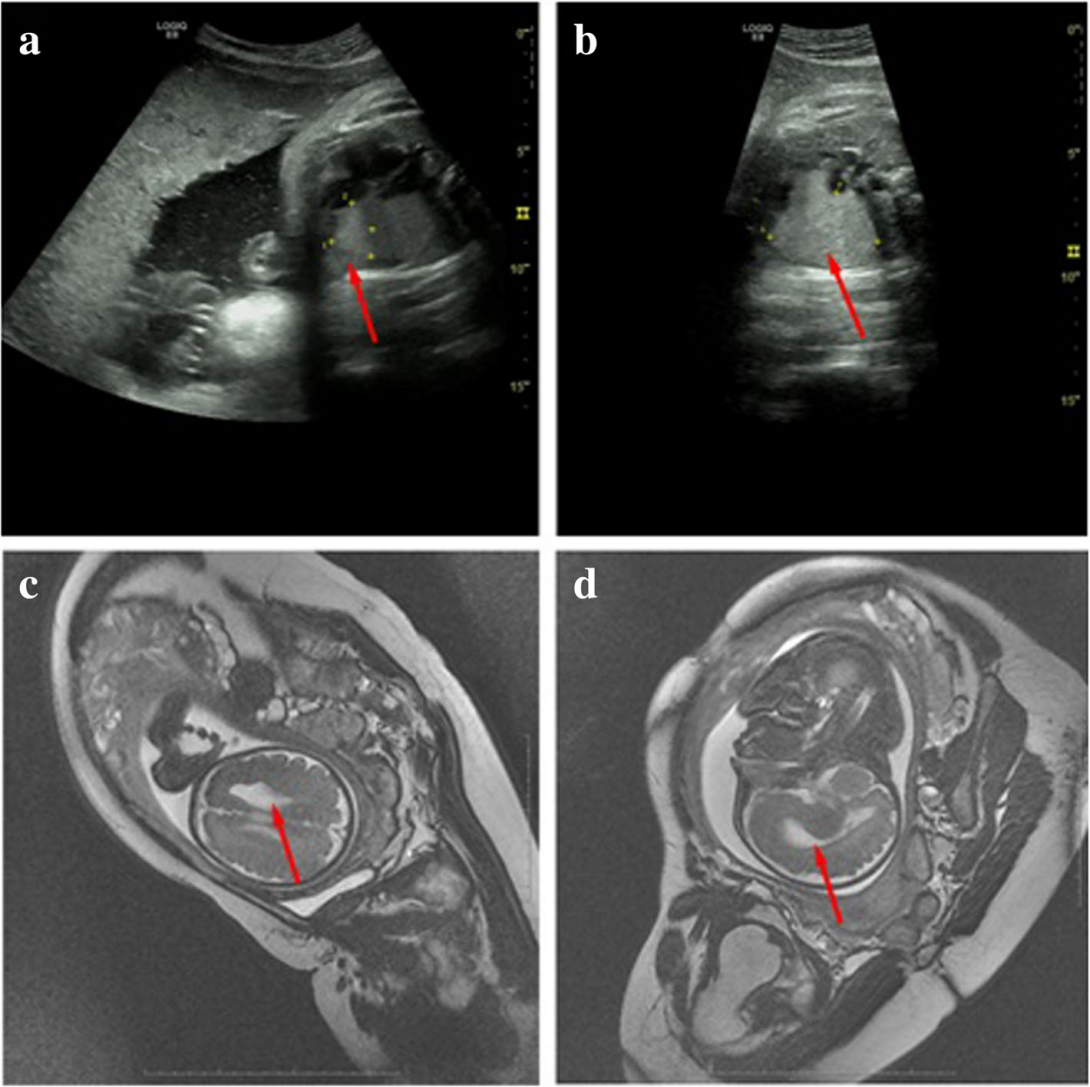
Color Doppler ultrasound and fetal brain magnetic resonance imaging examinations. a and b On January 25, 2018 (35 + 2 weeks of gestation), color Doppler ultrasound reexaminations of the fetal heart display multiple hyperechogenic masses (measuring 4.35 cm × 3.13 cm) in the fetal left ventricle, abnormal morphology of anterior horn of the left lateral ventricle with broadening of the internal diameter (2.02 cm); c and d on January 15, 2018 (34 weeks of gestation), fetal brain magnetic resonance imaging reveals abnormal morphology of the left frontal lobe with irregular extension of anterior horn of the left ventricle
